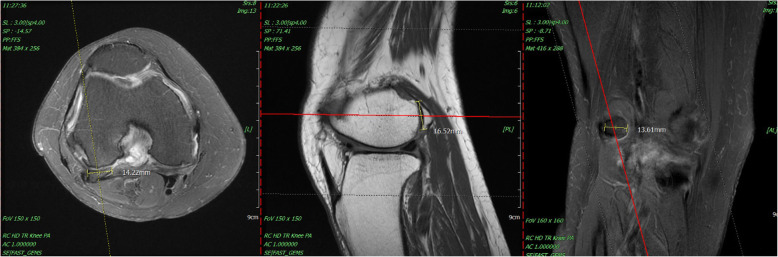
The right knee MRI showed a large fabella bone with subchondral bone edema and peripheral soft tissue effusion
